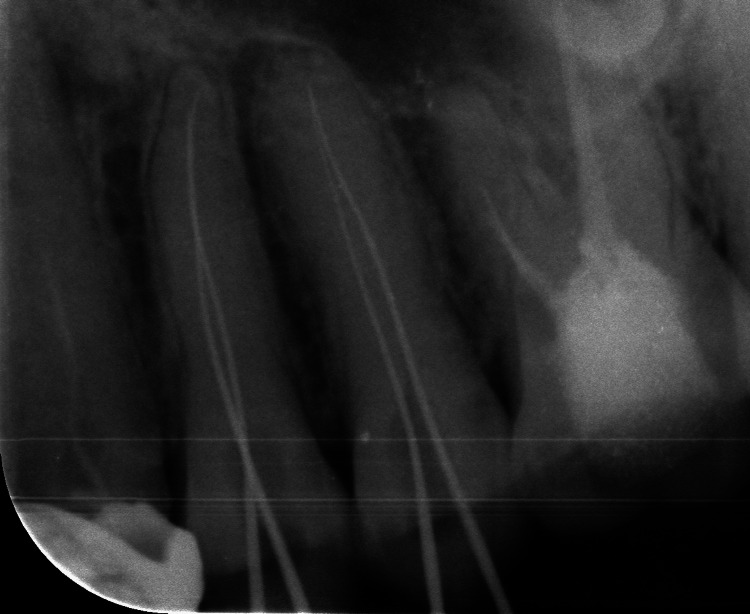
Working length measurement in 24 and 25 Radiograph showing working length measurement in 24 and 25 using a size 10 K file. The working length was found to be 21 mm
